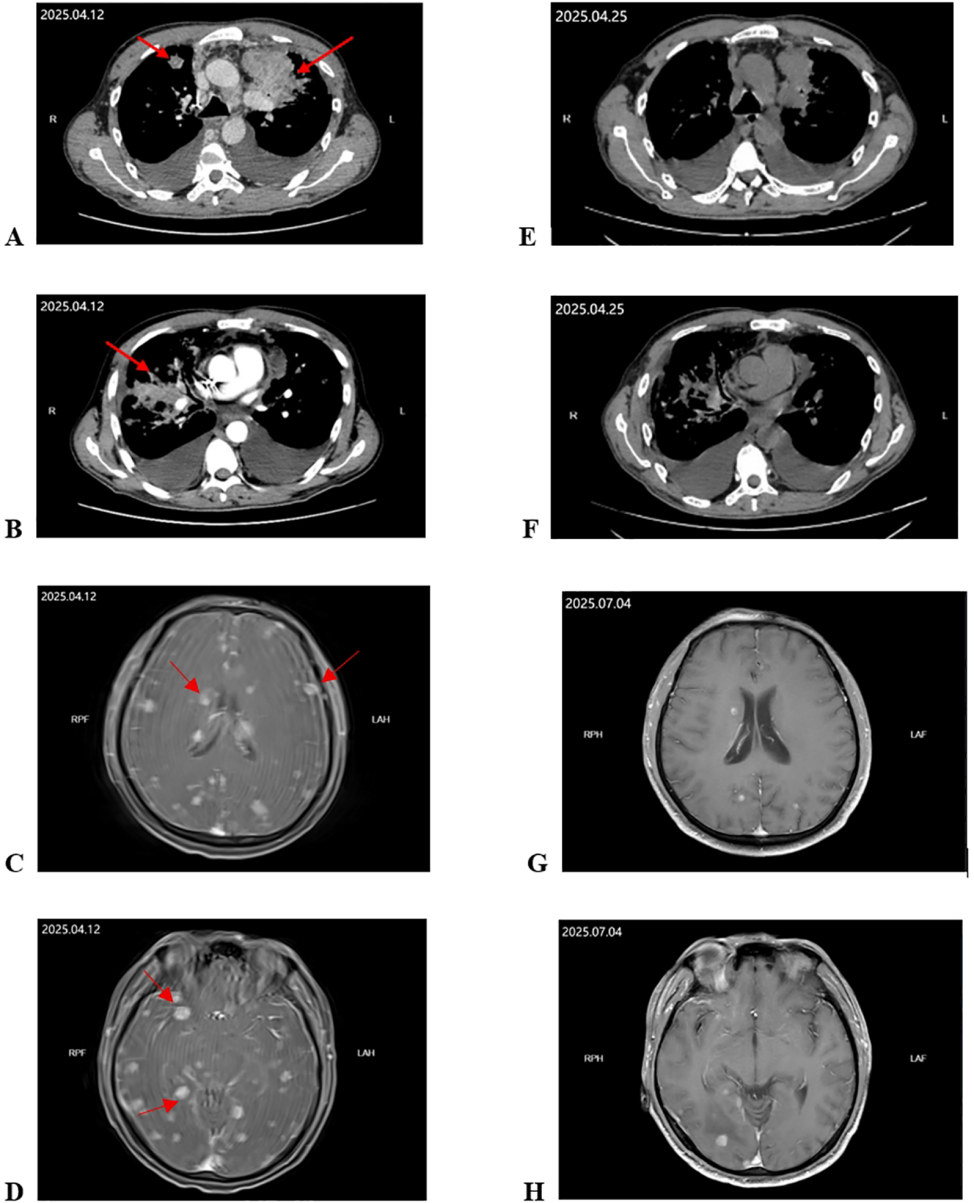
Changes in target lesion during treatment. (A–D) CT scan and MRI before treatment. (E, F) CT scan obtained 13 days after treatment. (G, H) MRI obtained nearly 3 months after treatment. The red arrows mark the target lesions.
